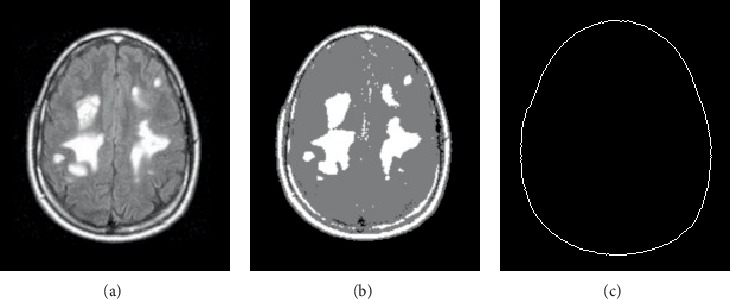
Applying *k*-means clustering on the MRI image and detection of Skull edge. (a) Original MRI image with tumors of different sizes. (b) *k*-means clustered MRI image. (c) Detection of the largest surrounding edge (skull).
